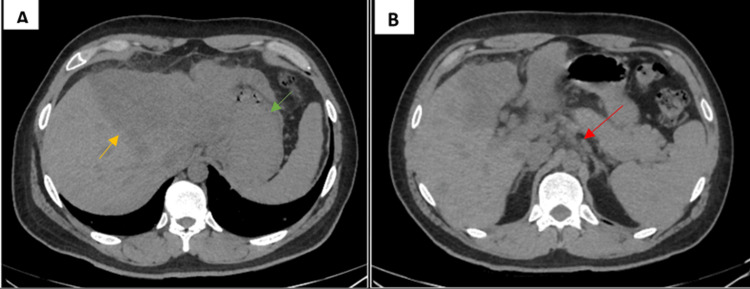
Abdominal CT demonstrated a low-density mass, involving the left hepatic lobe (green arrow) and medial segment of the right lobe (yellow arrow). B. The tumor exhibited celiac trunk adenopathies (red arrow). CT: computed tomography
